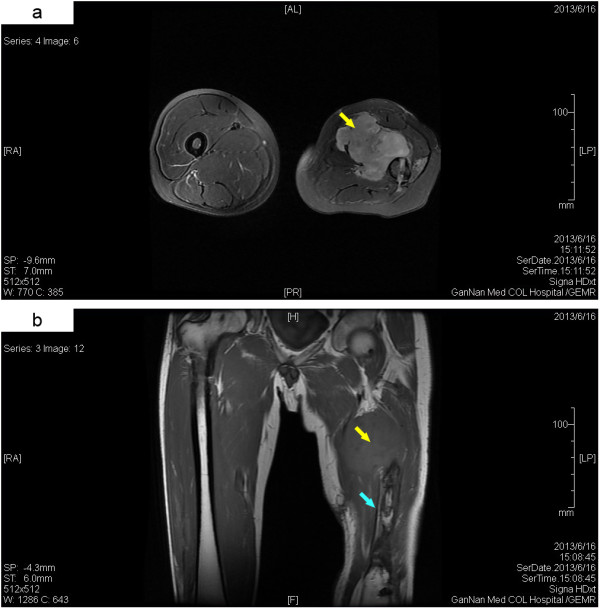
MRI images of the thigh. (a) Transverse T2-weighted image. (b) Coronal T1-weighted image. MRI images showed an irregular soft tissue mass with ill-defined margin in the anteromedial left thigh (yellow arrow), accompanied by surrounding soft tissue swelling and normal muscle structure disappearance. Bone destruction in the left femur shaft was observed (light blue arrow).
